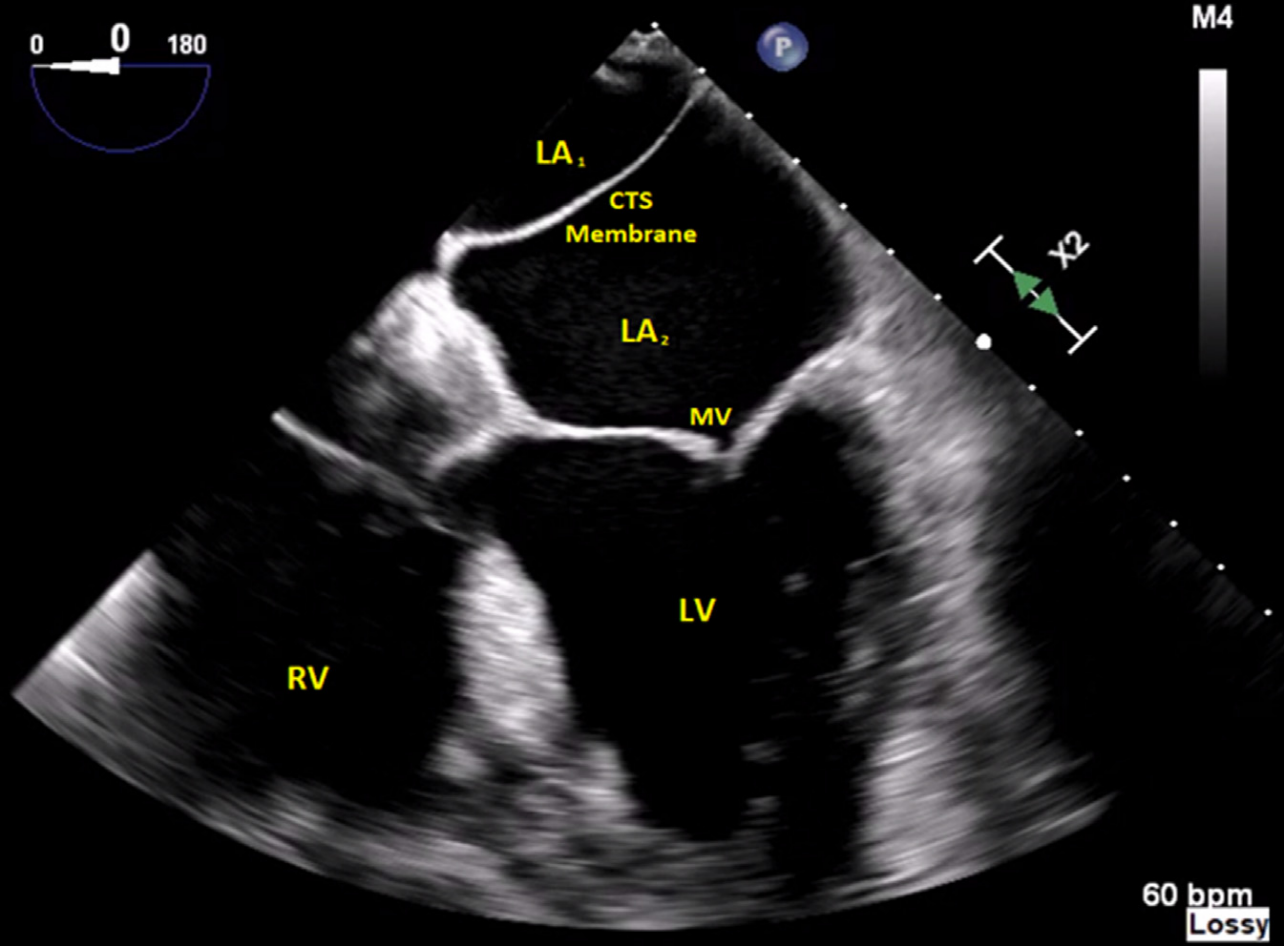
Transesophageal Echocardiography Revealing an Interatrial Membrane Consistent With Cor Triatriatum Sinistrum CTS = cor triatriatum sinistrum; LA = left atrium; LV = left ventricle; MV = mitral valve; RV = right ventricle.
